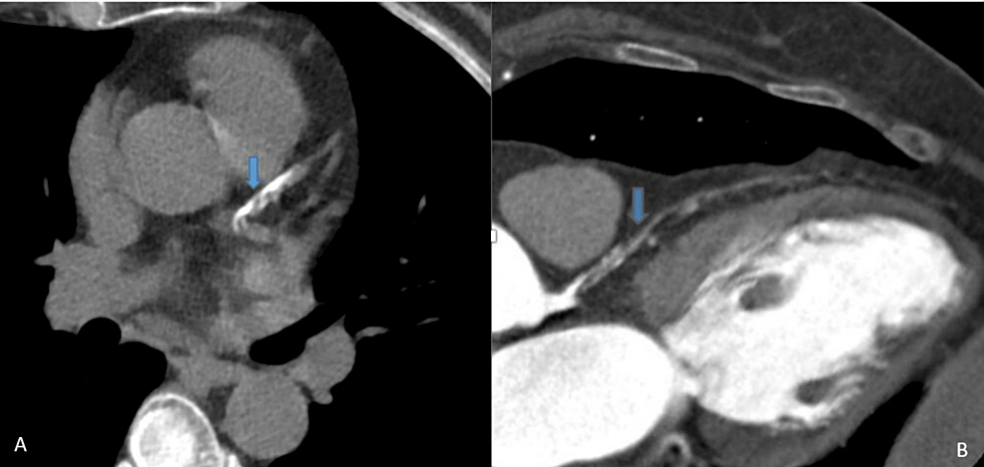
(A) Noncontrast CTCA and (B) contrast CTCA, showing a large calcified mixed density plaque/thrombus from left main bifurcation to the origin of LAD and to the origin of D1. Beyond that, hypoperfusion is noted in LAD (blue arrows) CTCA: computed tomography coronary angiography; LAD: left anterior descending artery
